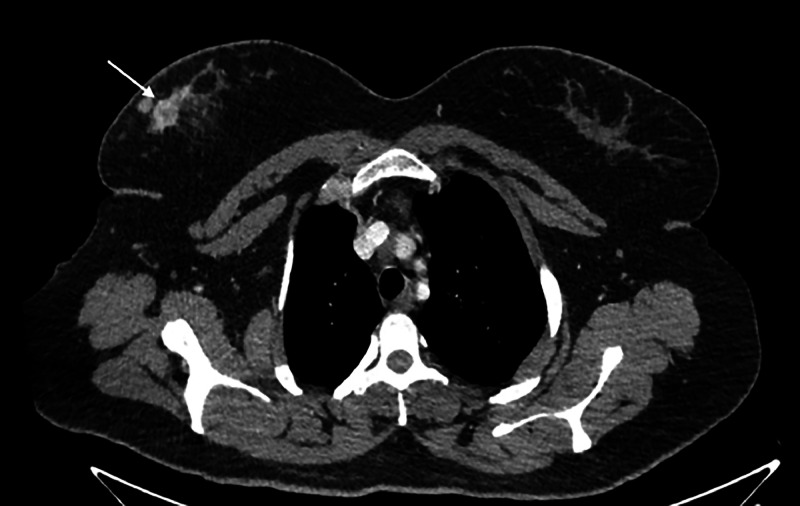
CT scan showing enhancing nodular mass (white arrow) in the upper outer quadrant of right breast with adjacent parenchymal distortion and subtle skin thickening. No distant metastasis.
